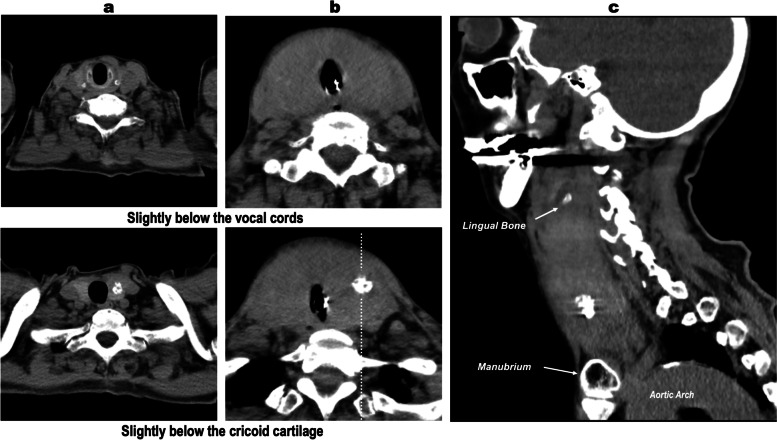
CT images of the thyroid gland a 3 weeks before fine-needle aspiration biopsy, b axial, and c sagittal views of the white dot line in b, immediately after tracheal intubation. a Three weeks before fine-needle aspiration biopsy. A mass with calcification is observed in the left lobe of the thyroid gland. The thyroid gland is hardly identified especially at slightly below the vocal cords. b Immediately after tracheal intubation. Diffuse swelling of the thyroid gland, especially at the subglottic level compared before biopsy. c Sagittal views of the white dot line in b. A swollen thyroid gland extending from the lingual bone developing beyond the manubrium of the sternum. No hemorrhage was observed
